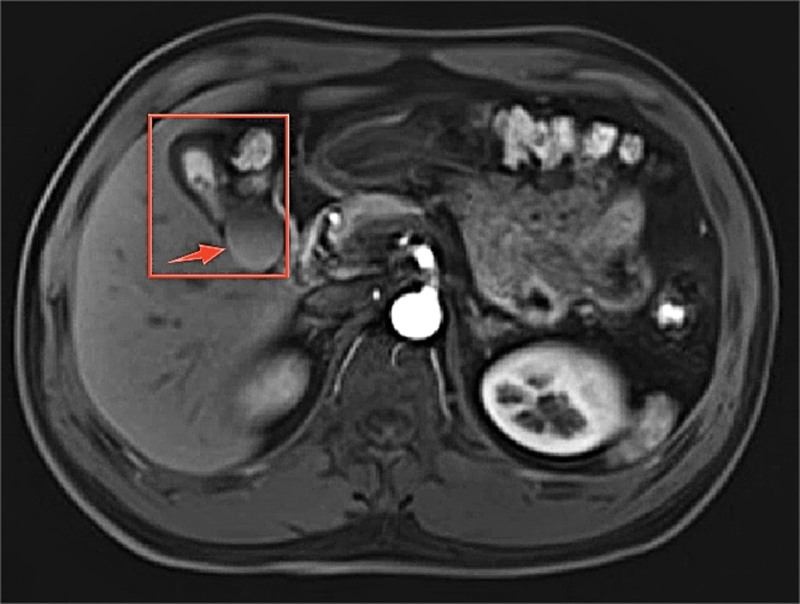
Preoperative MRI scan of the patient’s upper abdomen. The area within the red box is the cystohepatic triangle; the red arrow points to the cystic mass. MRI = magnetic resonance imaging.
